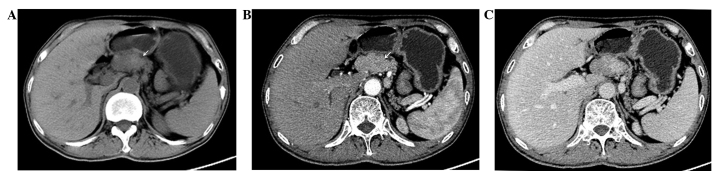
Computed tomography (CT) manifestations of glucagonoma syndrome. (A) Plain abdominal CT revealing an obscure mass (arrow) in the neck of the pancreas. The lesion was slightly enhanced during (B) the arterial phase and washed out during (C) the portal venous phase upon enhanced CT.
